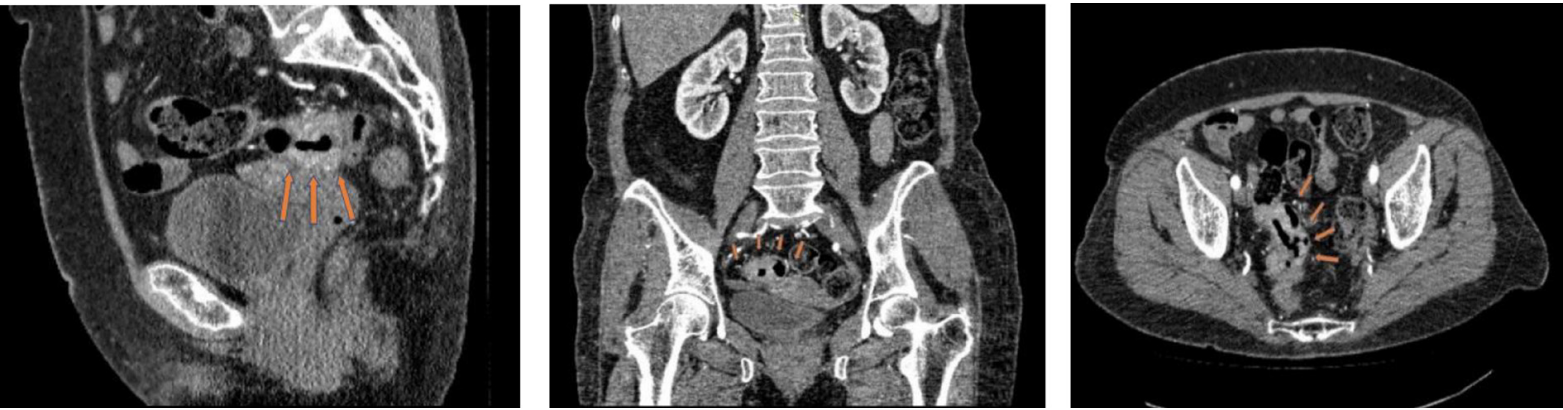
Pelvic CT colon with tumor, the arrows indicate tumor (a) sagittal projection, (b) anterior projection, (c) axial projection.
